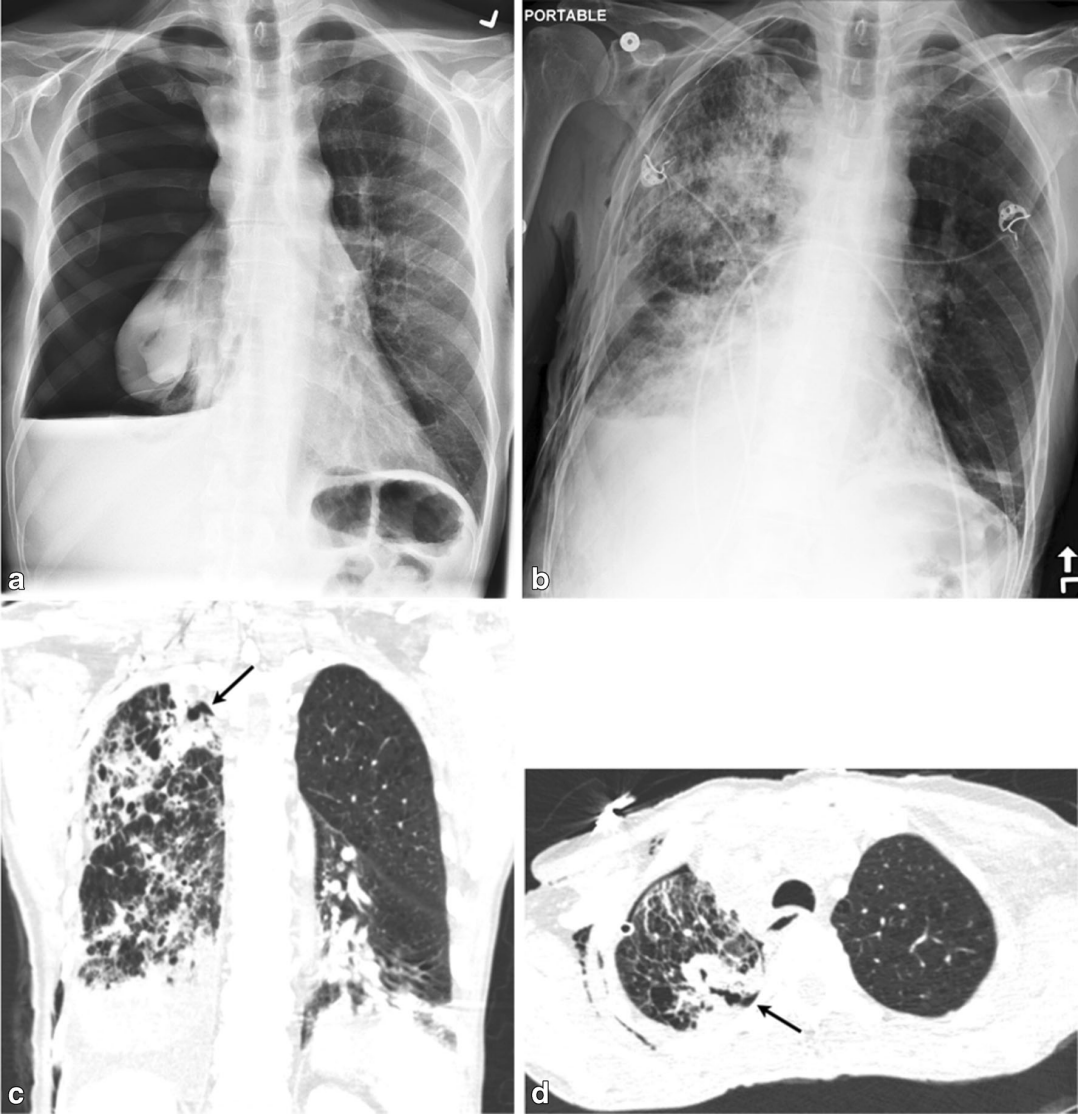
A 56-year-old male presented to the ED with right-sided chest pain. Frontal chest radiograph (a) demonstrated a large right hydro pneumothorax, which did not completely resolve after chest tube placement (b). Coronal (c) and axial (d) CT images demonstrated a cavitating cancer within the right upper lung with an associated alveolopleural fistula (*arrow*). In addition, right paratracheal lymphadenopathy is seen. Extensive alveolar opacities in the re-expanded lung are consistent with pneumonia and reexpansion edema
